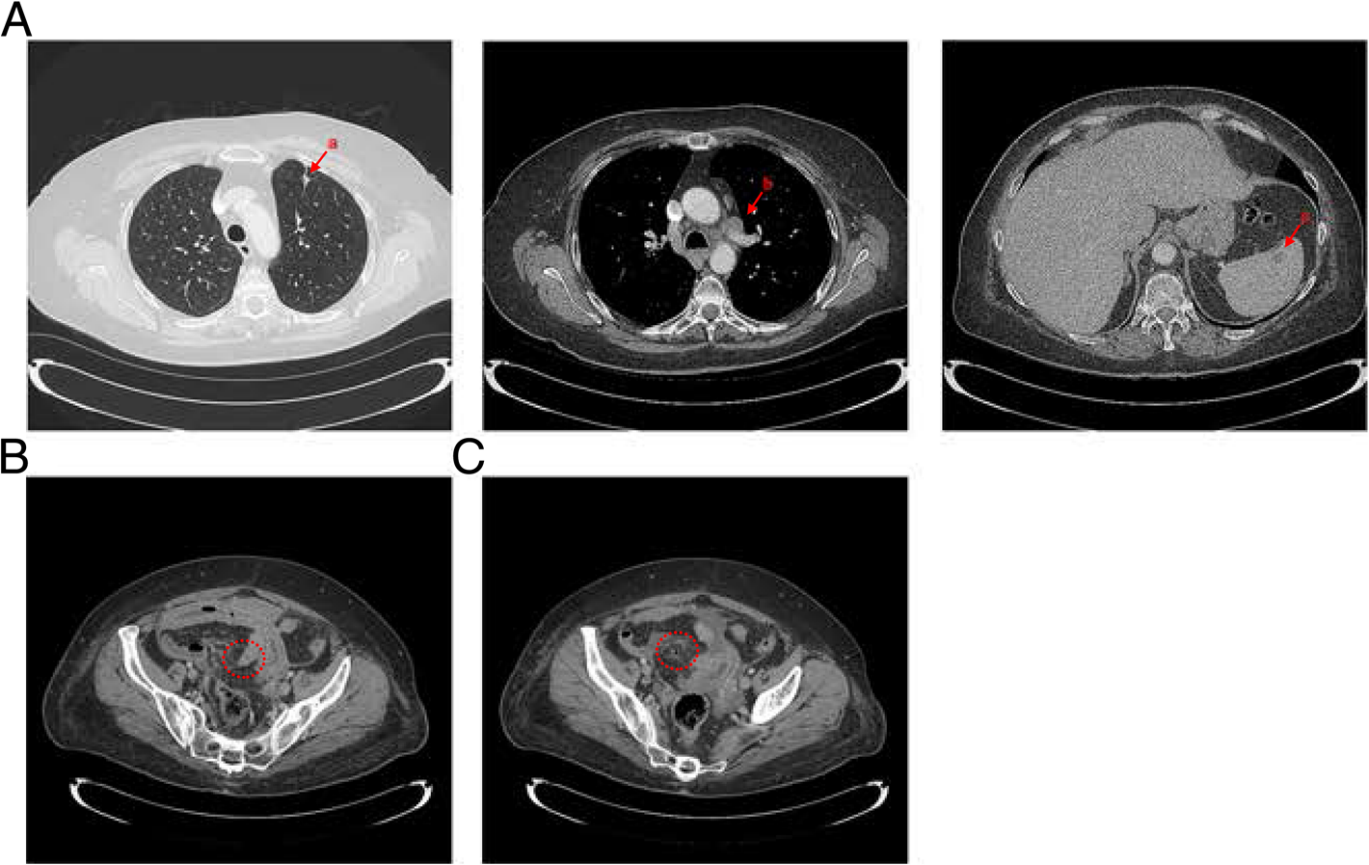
Post-treatment CT images of the lungs and spleen and abdominal images indicating small bowel perforation. a. Transverse CT images of the chest and abdomen showing significant reduction of the (*a*) anterior left upper lung lobe lesion, (*b*) left lung hilar lesion and (*c*) single splenic lesion. b. Transverse CT image showing submucosal edema (“target sign”) of the small bowel (red circle). c. Transverse CT image showing foci of non-dependent extraluminal air adjacent to the bowel (red circle)
